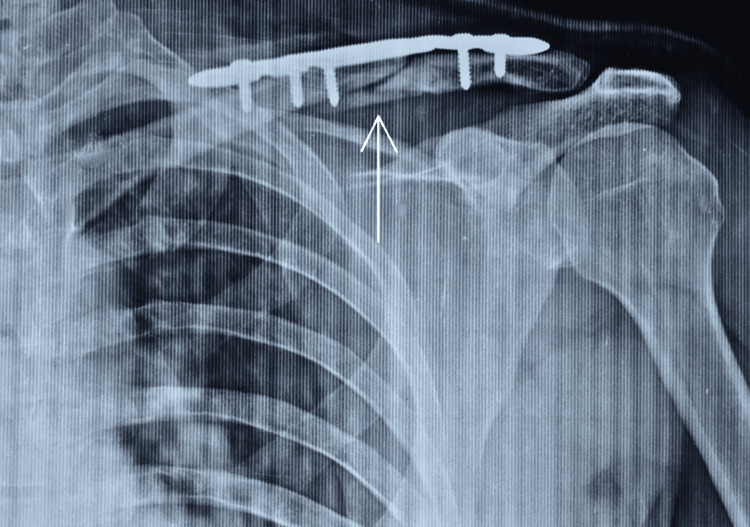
X-ray taken nine months post-operatively depicting absence of bridging callus (arrow).
